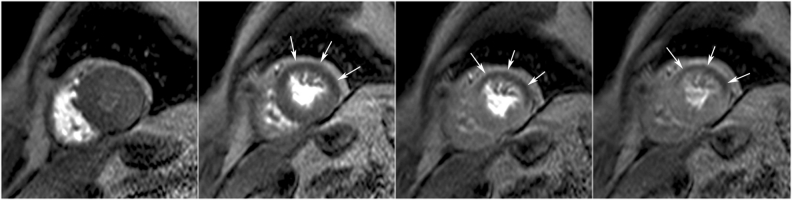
MRI stress perfusion study in a 73 old year female with suspected mid LAD coronary artery in–stent stenosis. Midventricular short–axis serial time frames of “first pass” perfusion during dipyridamole vasodilatatory stress show Gd contrast successively enhancing the right, left chambers and myocardium (*images from left to right*). A transmural perfusion defect in the anterior and lateral walls is seen (*arrows*). The patient was referred for catheterization which confirmed a 90% in–stent stenosis and was treated by PCI with drug eluting stent placement.
